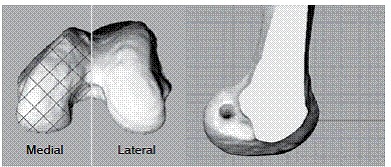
Rendered 3D CT protocol scan using Rhinoceros^(r)^ software. (A) Axial view, with most superior intercondylar notch plane set. (B) Lateral view after medial condyle was cropped, showing central point of ACL tunnel (green dot) at the medial wall of the lateral condyle.
